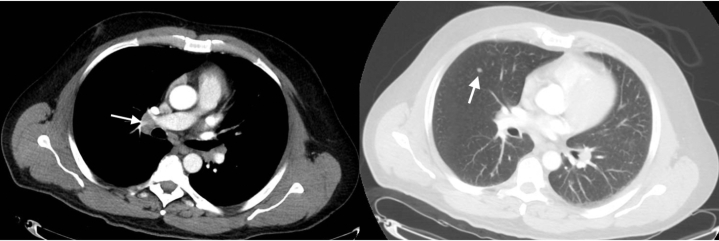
A, B, Trauma protocol CT angiography of chest. A, Mediastinal window with mild mediastinal and hilar lymphadenopathy (arrow – right level 10 lymph node). B, Lung window with 5-mm right major fissure nodule (arrow).
